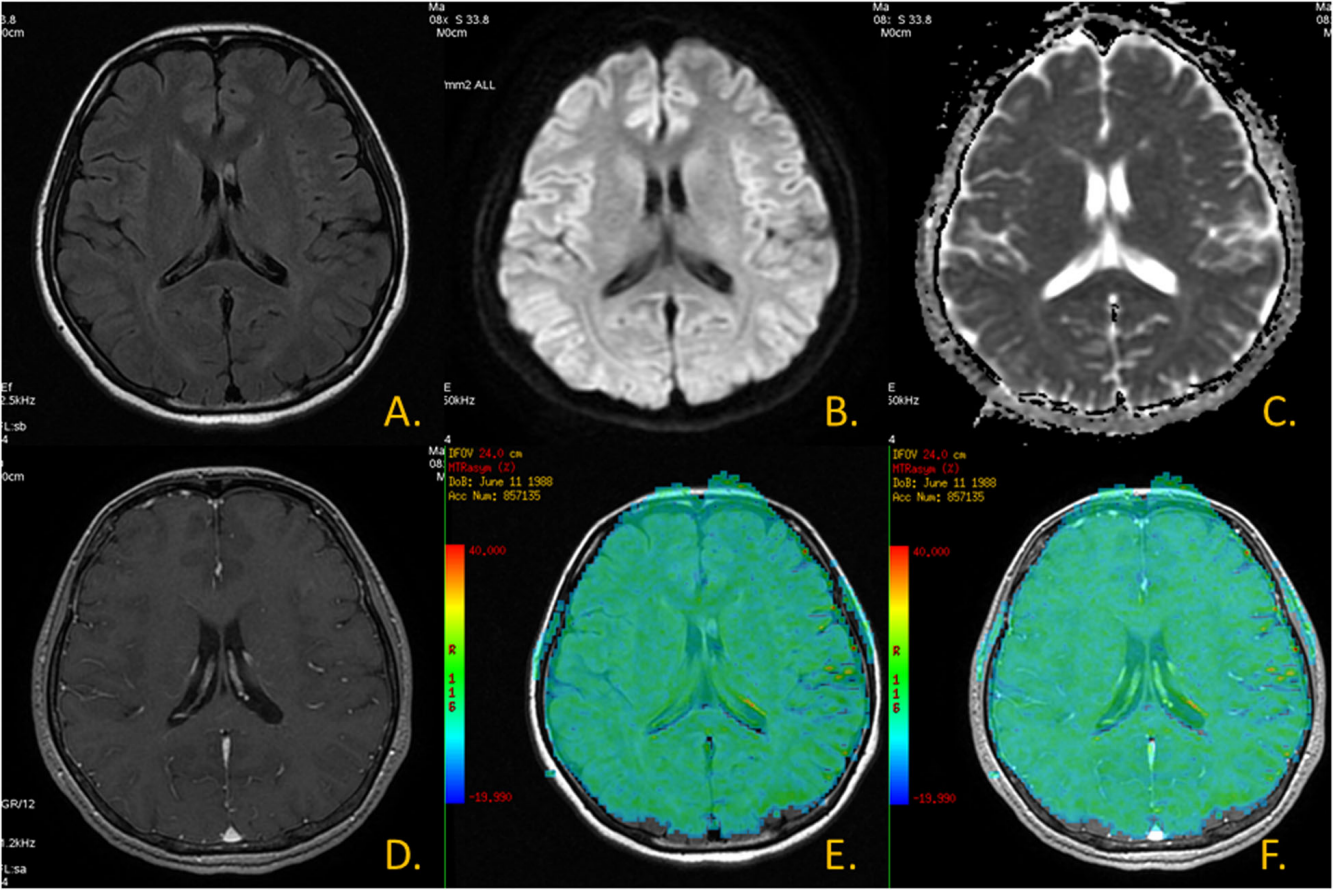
Normal volunteers. a.T2 FLAIR image; b.DWI; c.ADC map; d.T1WI + C; e. CEST MTR map and T2 FLAIR image fusion; f. CEST MTR map and T1WI + C fusion
